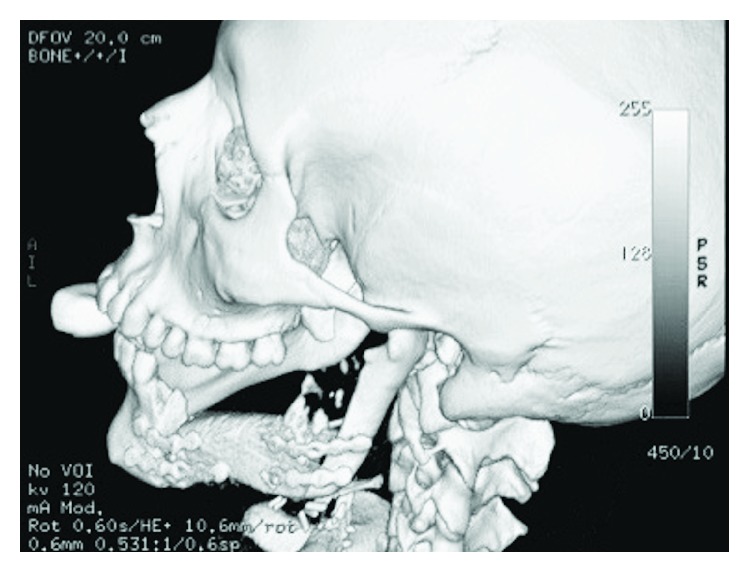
Radiographic image after reconstruction by FRF.
